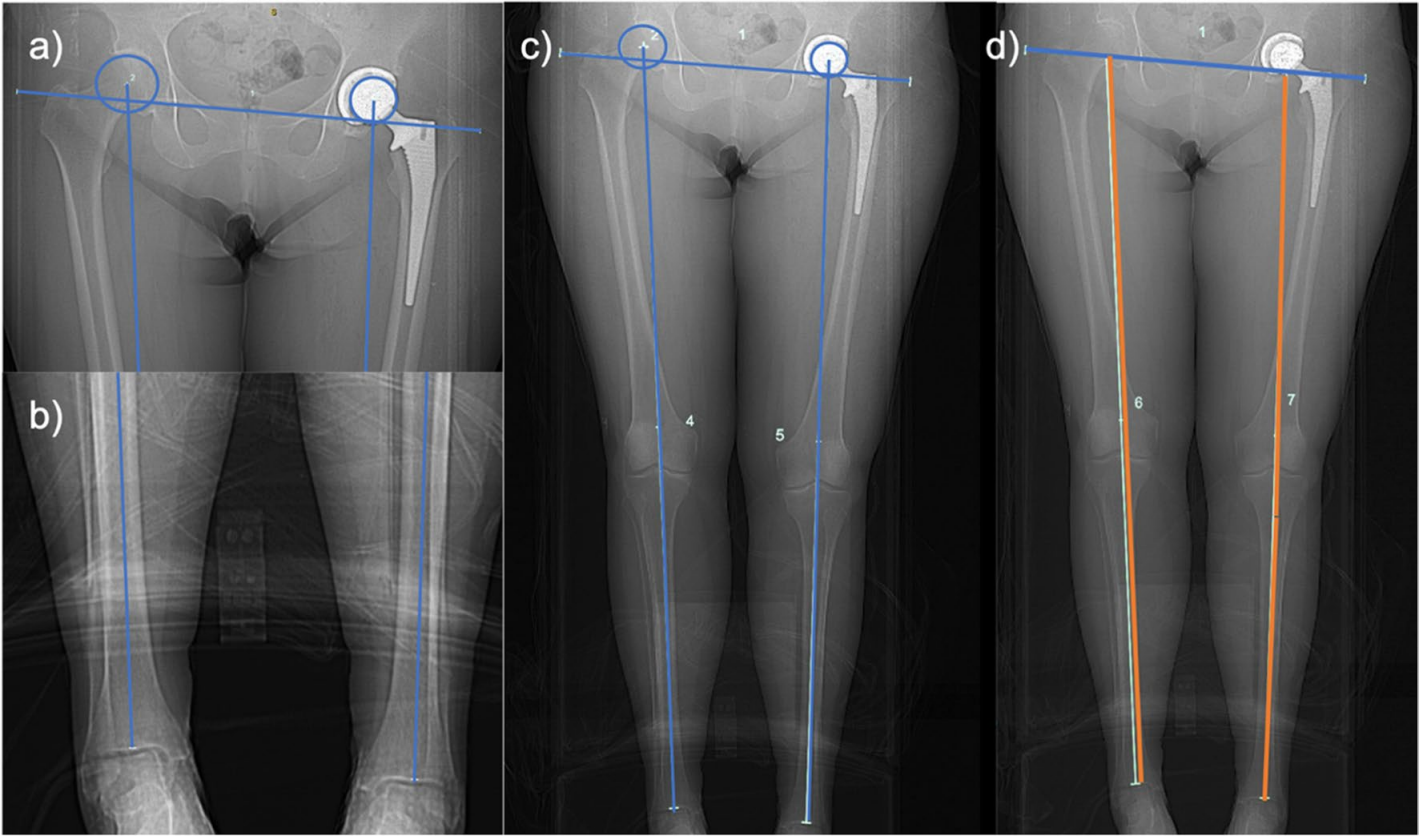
CT scanogram image demonstrating measurement of inter-teardrop to tibial plafond distance (IT-TP). a The centre of the native or prosthetic femoral head was defined and a transverse line was drawn passing through the most inferior point of each pelvic teardrop. b The centre of the tibial plafond was defined. c The mechanical axis of each limb was then drawn between the centres of the femoral head and tibial plafond. d The IT-TP (orange line) was defined as the distance between the centre of the tibial plafond and the intersection point of the mechanical axis and inter-teardrop line
